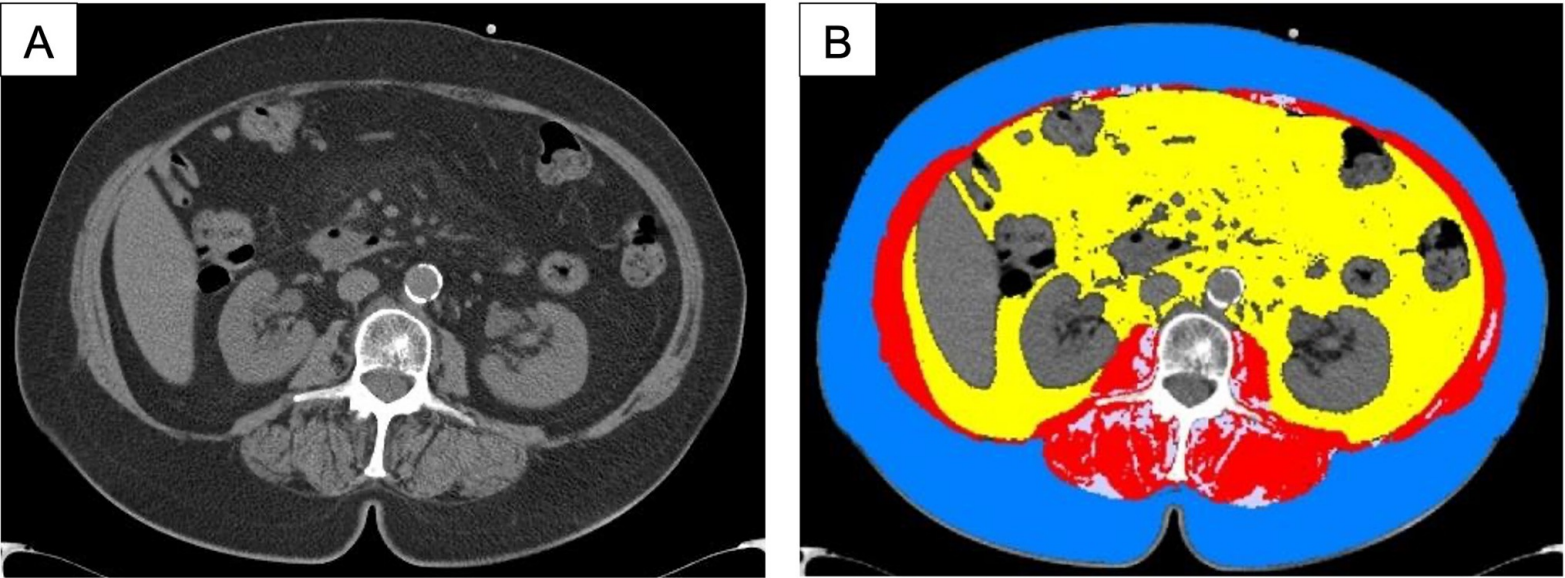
Axial CT-scans of patient with pancreatic ductal adenocarcinoma, performed at the L3 vertebral body level. BMI was increased with 27.7 kg/m^2^, SMI was decreased with 30.3 cm^2^/m^2^.DC A—initial CT image; B—segmented CT scan. The area of muscle tissue was 87.5 cm^2^, the total area of fat tissue—481.8 cm^2^.
